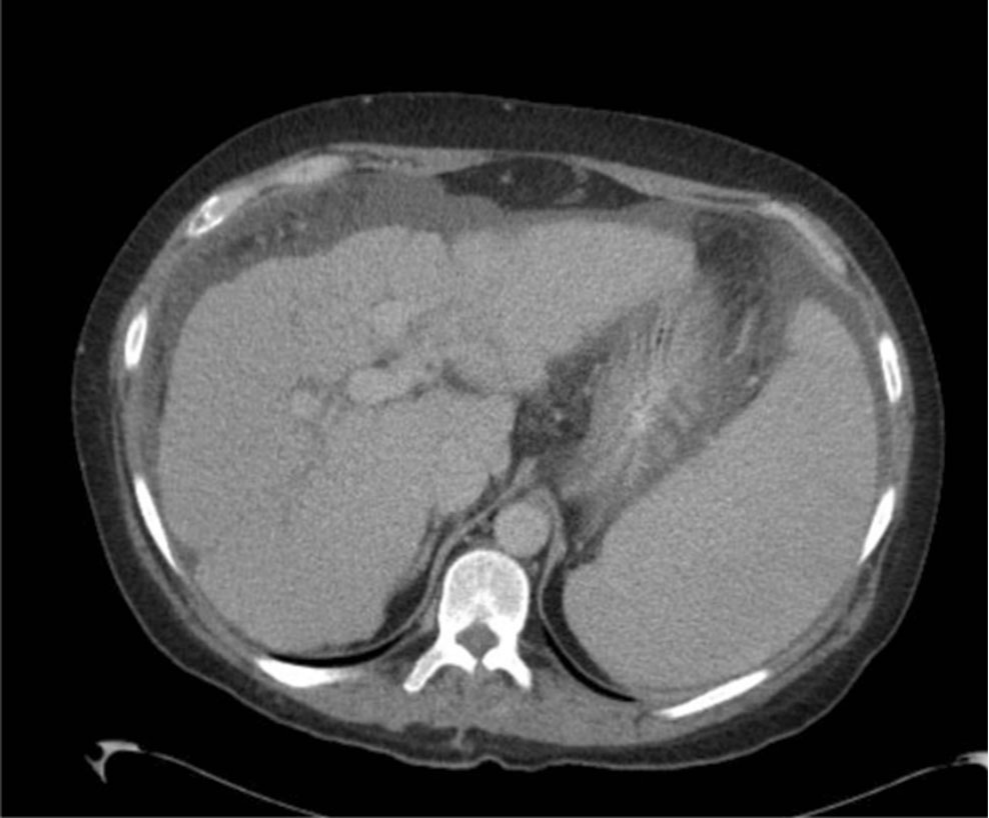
Portal venous CT demonstrates an irregular nodular liver, with the capsule outlined by ascites. Note splenomegaly due to portal hypertension
